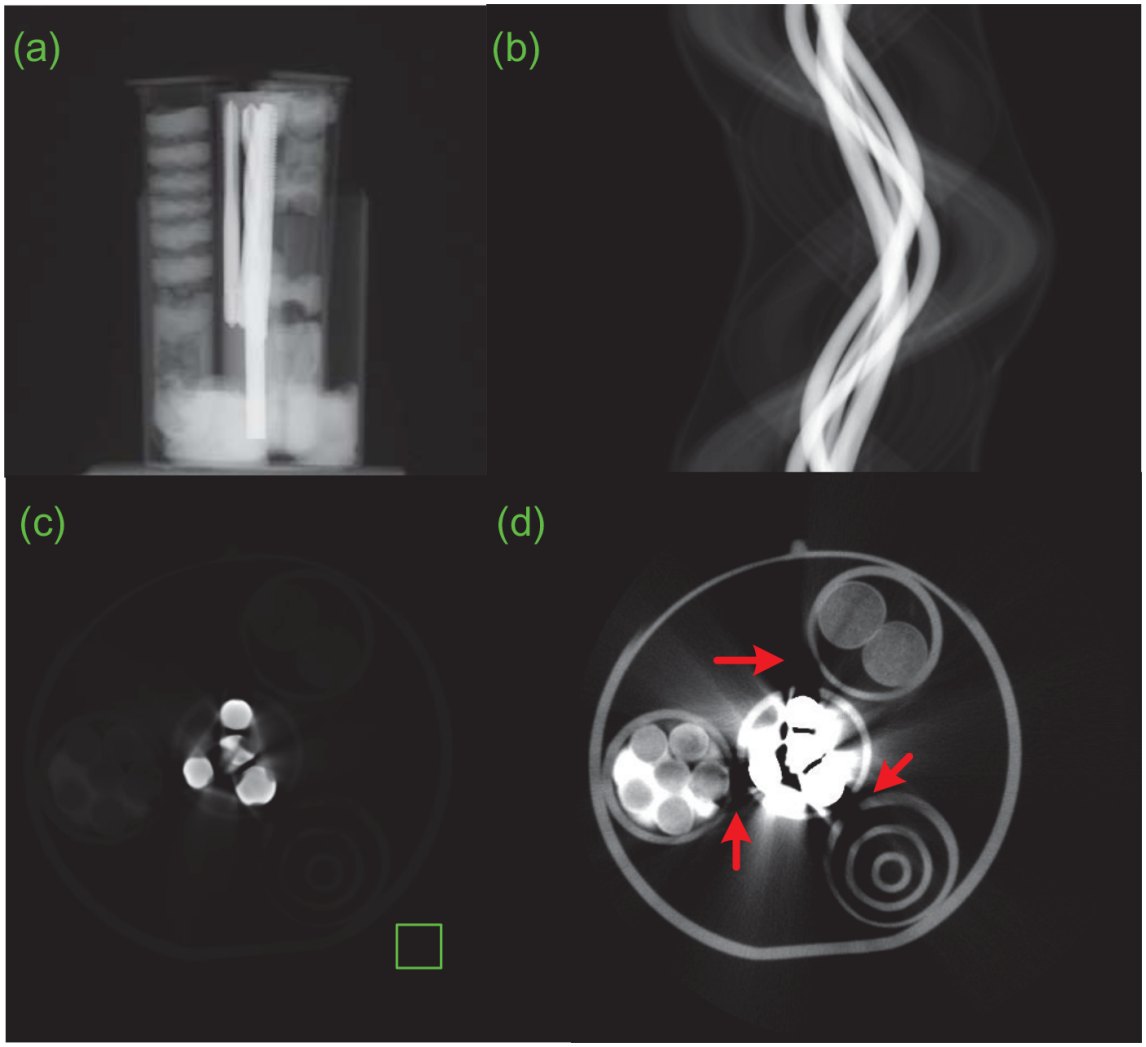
The conventional 3rd CT experimental results. (a) is one of the two-dimensional projection images, (b) the projection sinogram and (c) the reconstructed images with ART in Eq (1). (c) is displayed into (d) by histogram equalization.
